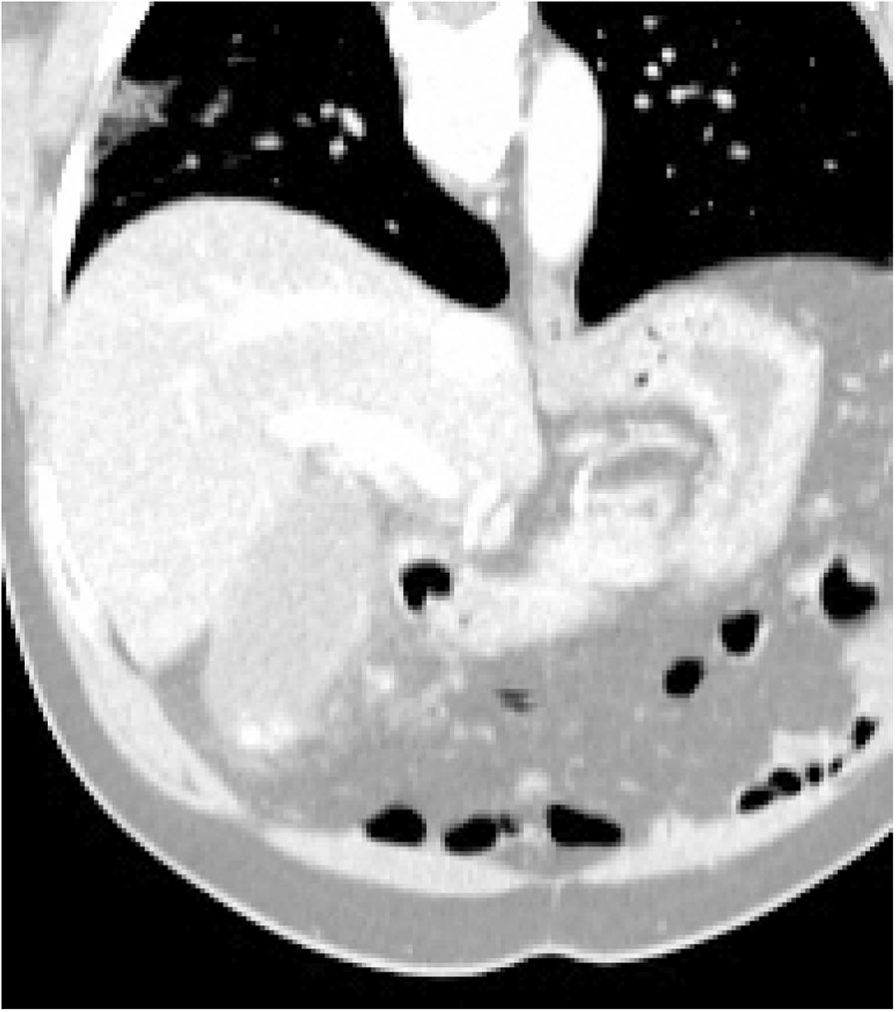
Abdominal CT scan. The image shows fat infiltration around a distended gallbladder that has wall thickening and stones, as well as a subsegmental ground glass opacity in the right lower lobe
